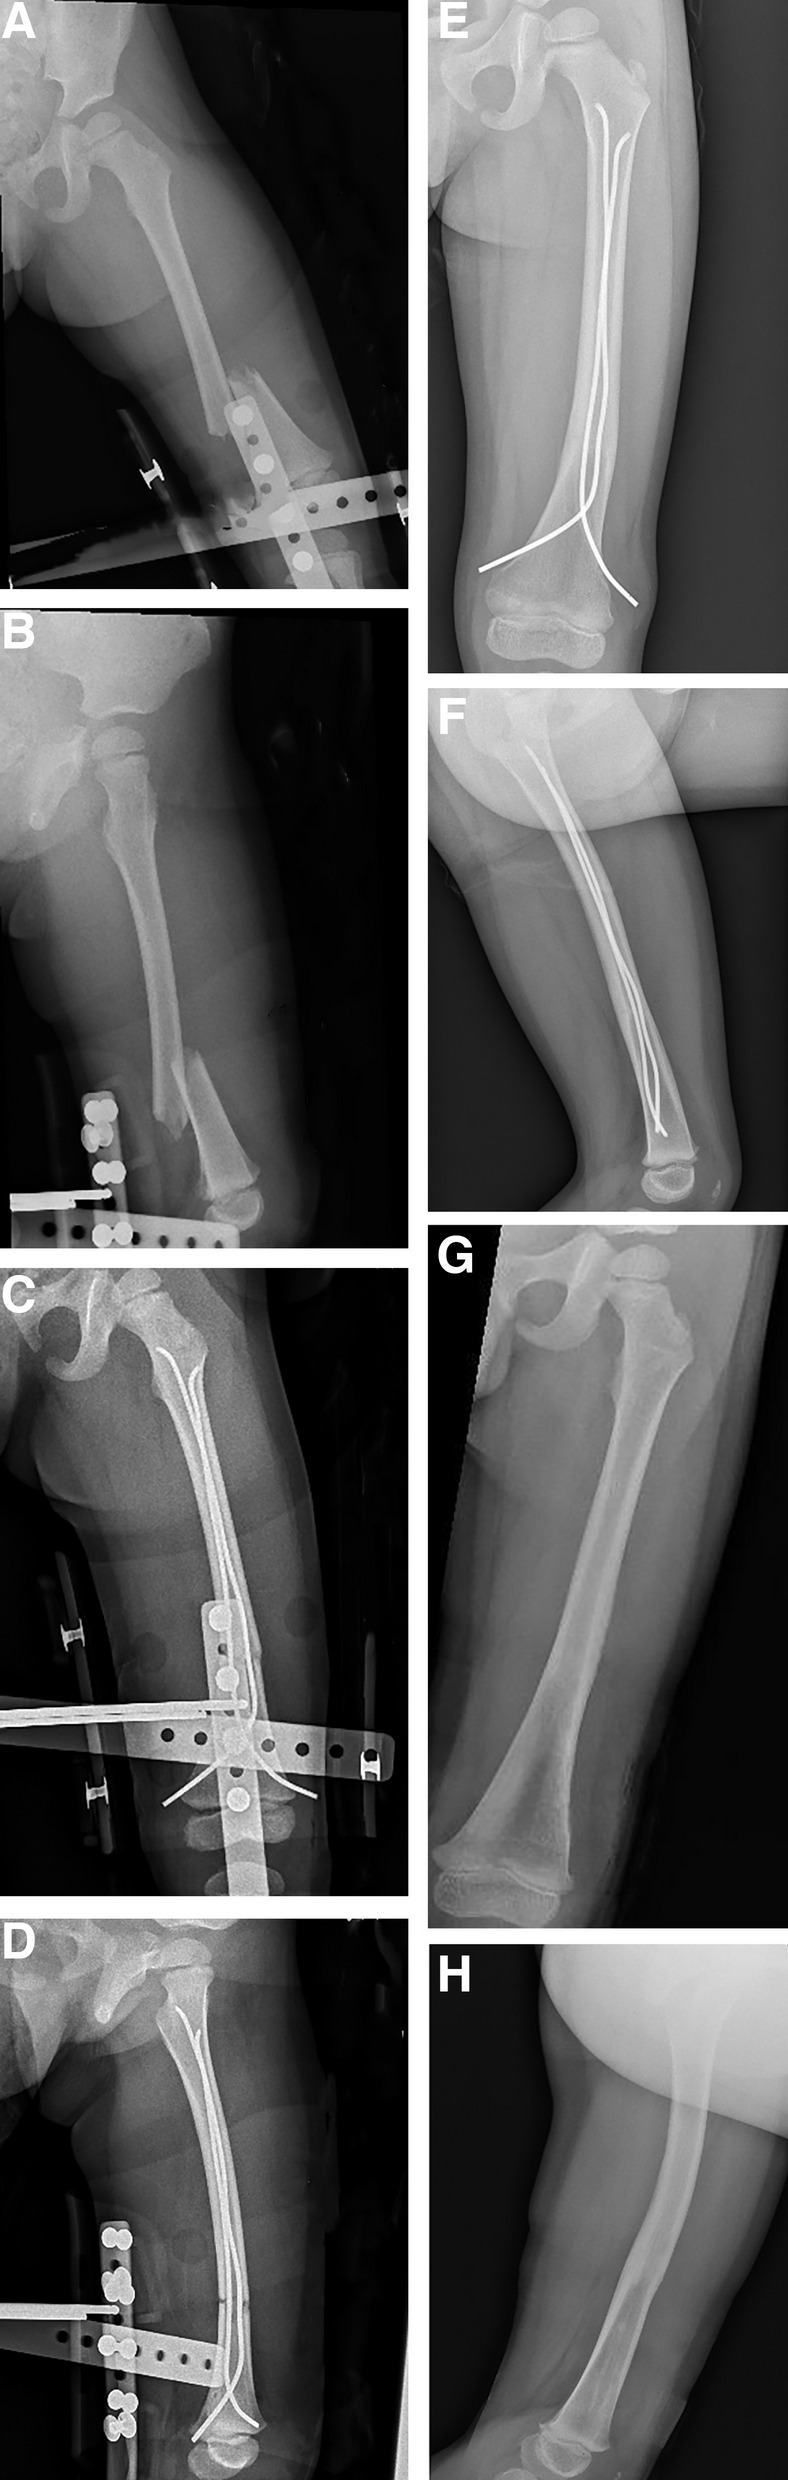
A 4-year-old girl with brace fixation in emergency department, with X-ray indicating MDJ fracture as a result of motor vehicle accident (A, B); the girl received ESIN treatment, and the intraoperative reduction was satisfactory (C, D). Additional brace was adopted to compensate for instability. With the fracture union after 6 months (E, F), the implant was removed under general anesthesia after 10, the knee activity and lower limb function were normal at the 13-month follow-up (G, H)
